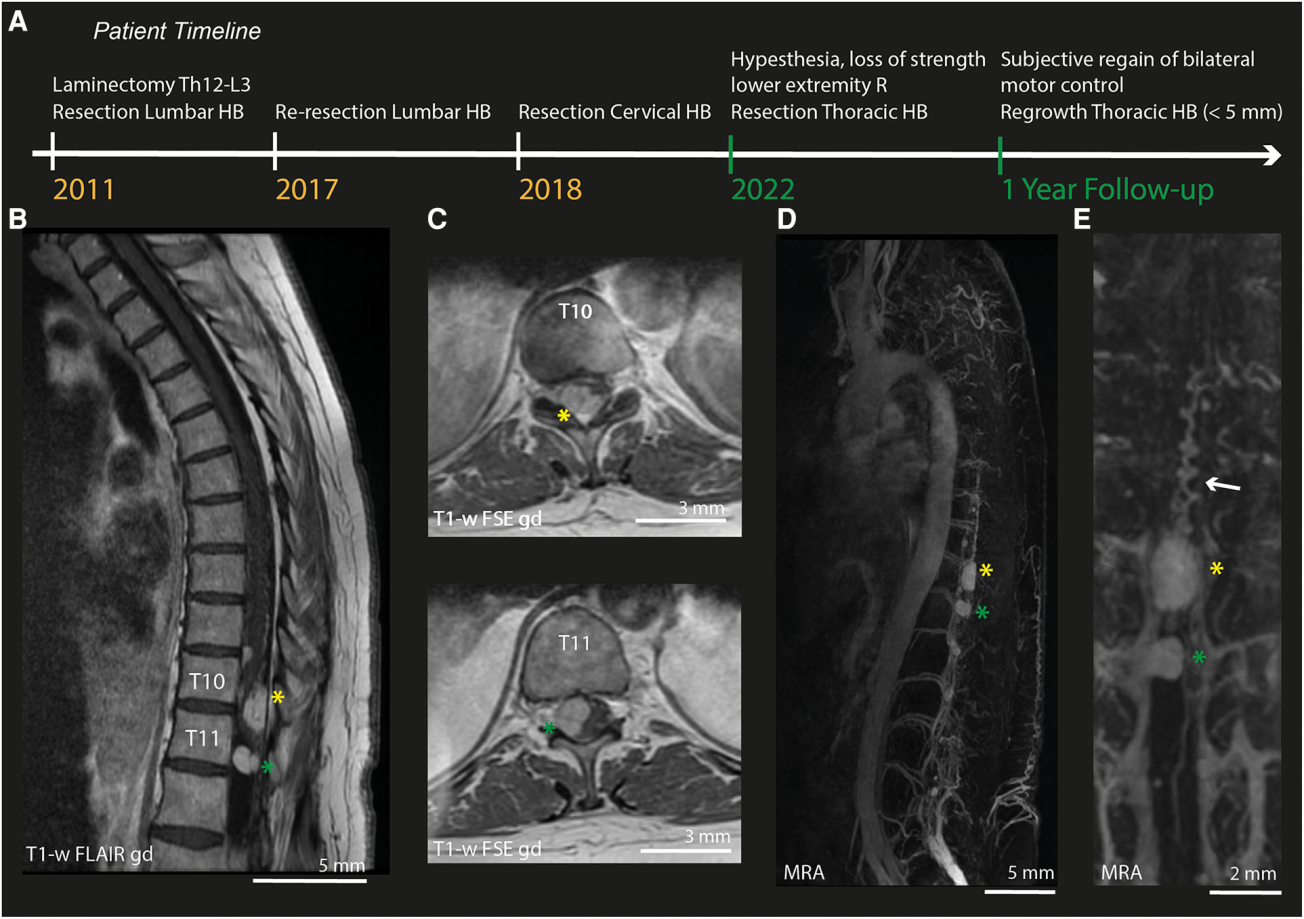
Pre-operative MRI and MRA. (A) Timeline of relevant surgical treatments undergone by the patients described in this case. Green labels indicate events during this episode of care. (B) Sagittal MRI-section of the thoracic spinal cord (T1-weighted FLAIR after gadolinium). (C) Axial MRI-slices (T1-weighted FSE after gadolinium) of lesion 1 at T10 (*yellow asterix*) and lesion 2 at T11 (*green asterixis)*. (D) MRA of the full spinal cord. (E) Zoom in on the MRA of both lesions. HB, Hemangioblastoma; R, Right; T1-w, T1-weighted; gd, gadolinium; MRA, Magnetic Resonance Angiography.
